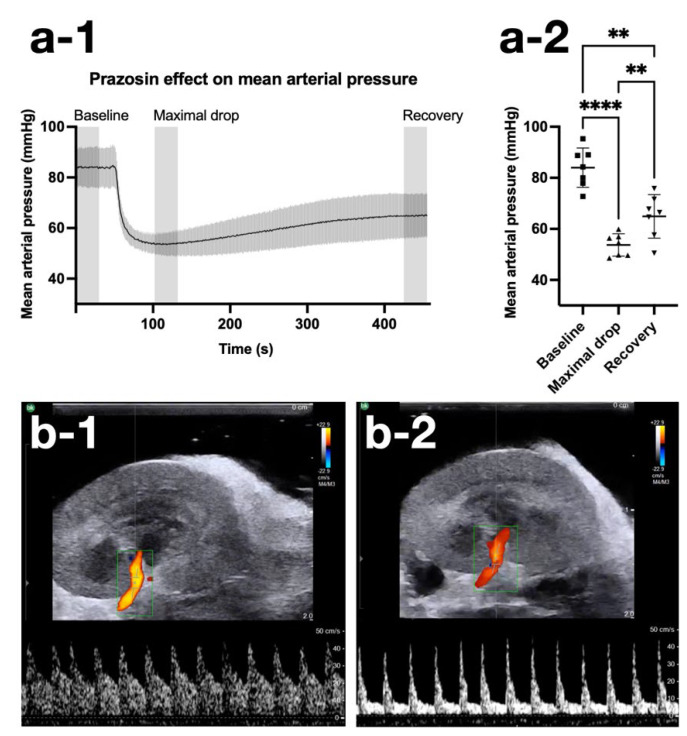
Effect of prazosin on mean arterial pressure and renal artery triplex Doppler. (a-1,a-2) show the effect of prazosin on mean arterial pressure during SRUS scan 2 (mean and standard deviation shown). (b-1) shows an example of the color Doppler and spectral Doppler wave pattern from the normal renal artery or one of its branches at baseline before SRUS scan 1. (b-2) shows a decreased color Doppler signal and altered spectral Doppler wave pattern from the same artery after completion of SRUS scan 3.  = *p* ≤ 0.01,  = *p* ≤ 0.0001.
